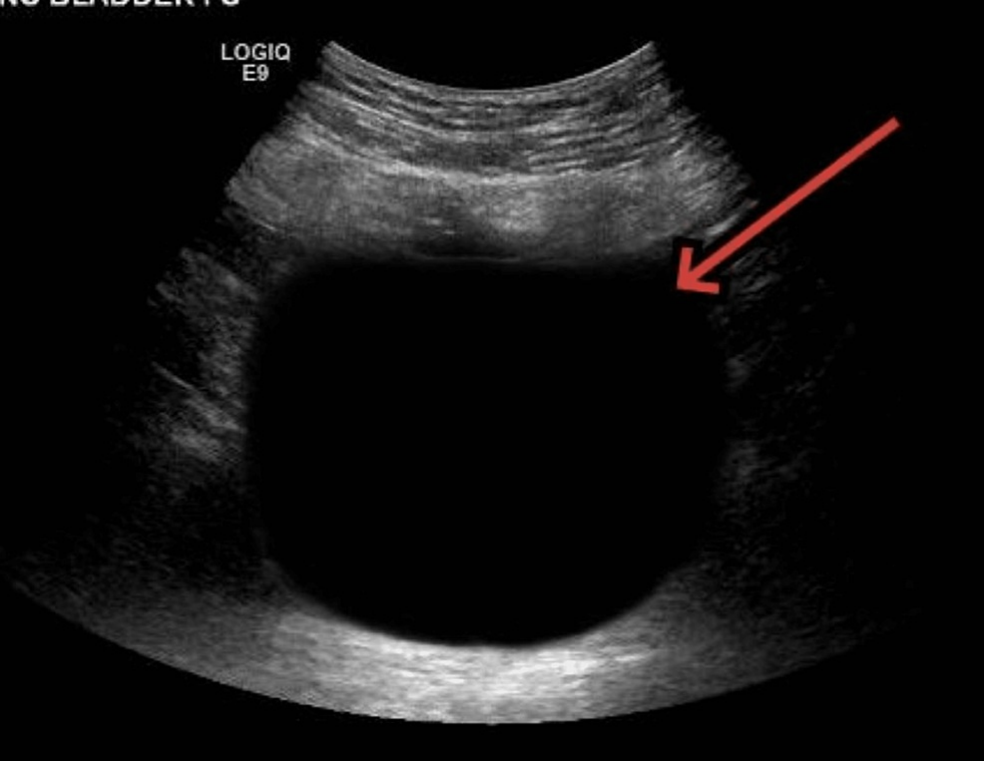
Bedside ultrasound image of the urinary bladder. The red arrow points toward the distended urinary bladder.
